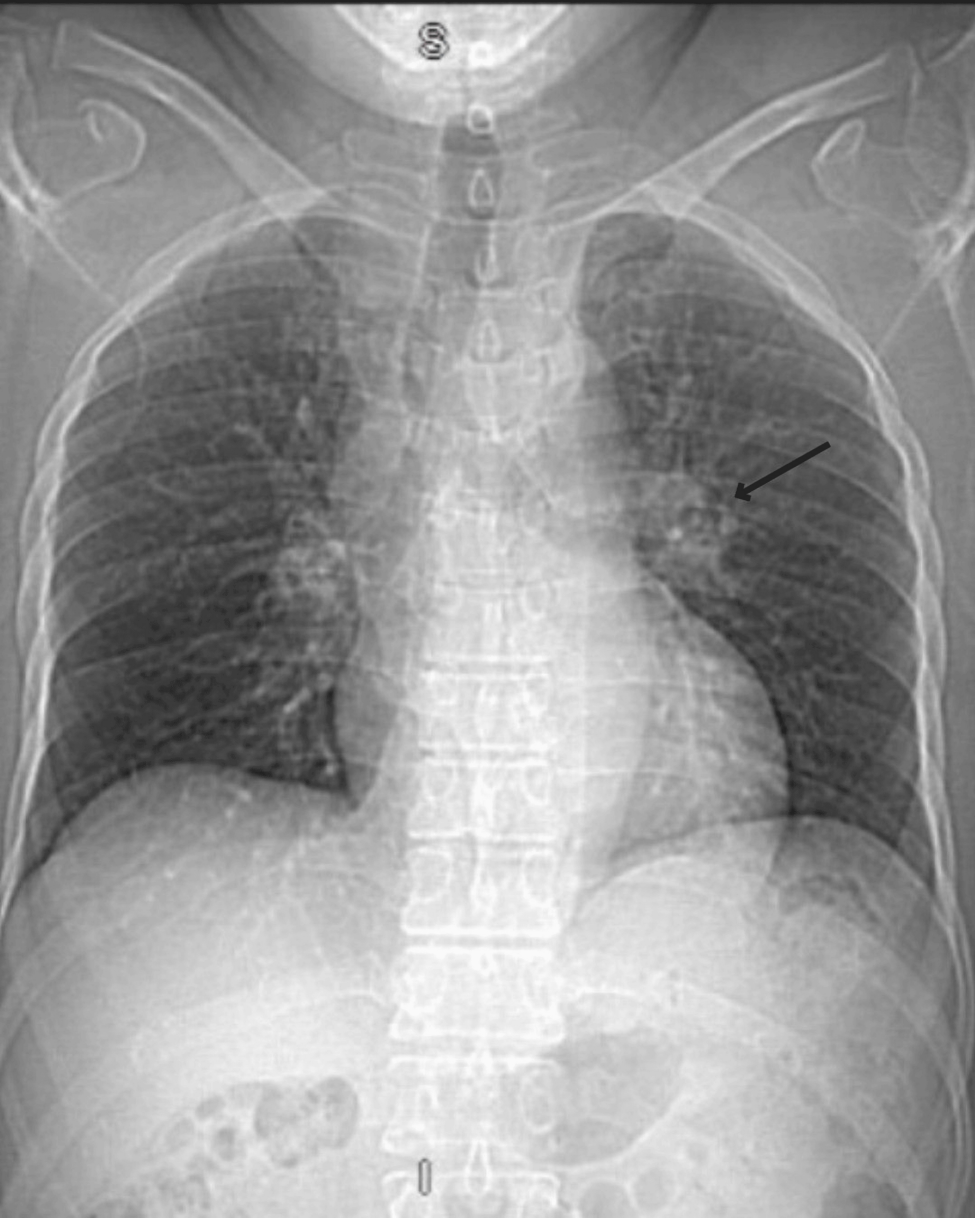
Chest X-ray showing ground-glass lesion around the left hilar region
